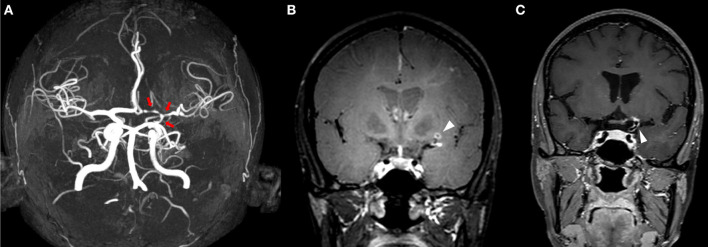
MRI of a patient presenting with a secondary ACNS in the context of systemic lupus erythematosus. Time of flight-MRA showing stenotic lesions in the terminal part of internal left carotid, middle and anterior left cerebral arteries [(A) red arrows]. IVW-MRI showing multiples areas of concentric contrast enhancement in the terminal part of internal carotid and in the middle artery in the left side [(B,C) arrowheads].
